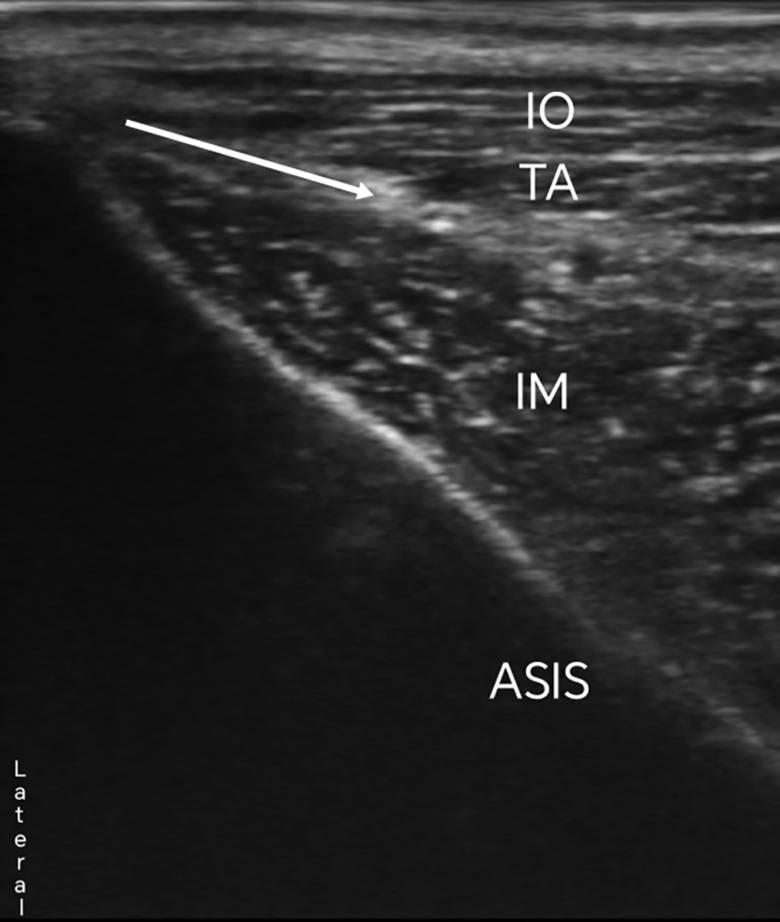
Ultrasound-guided supra-inguinal fascia iliaca compartment block. Transverse ultrasound image obtained with the probe placed at the level of the anterior superior iliac spine. Labeled structures: tensor fasciae latae muscle (TFLM), lateral femoral cutaneous nerve (LFCN), and sartorius muscle (SaM).
